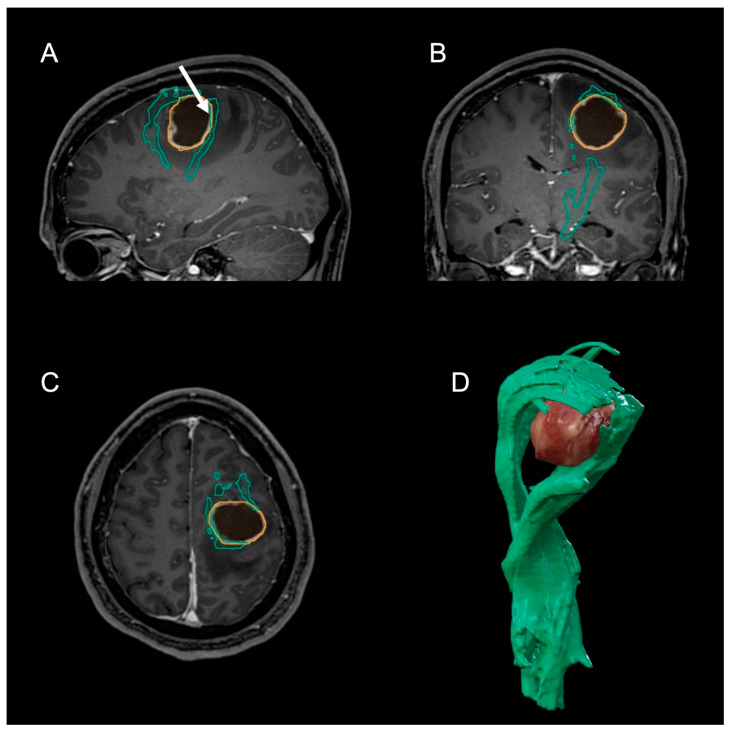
Preoperative MRI in T1-weighted sequence with contrast enhancement (A–C). Arrow: location of positive intraoperative DES at 2 mA. Note the cystic tumor (orange outlines) within the left precentral gyrus. Green outlines: CST. (D) Preoperative tractogram of the left CST (green) suggests fibers ventral and dorsal from the tumor. Intraoperative DES revealed primary motor neurons only dorsally. (MRI: magnetic resonance imaging; CST: corticospinal tract; DES: direct electrical stimulation).
